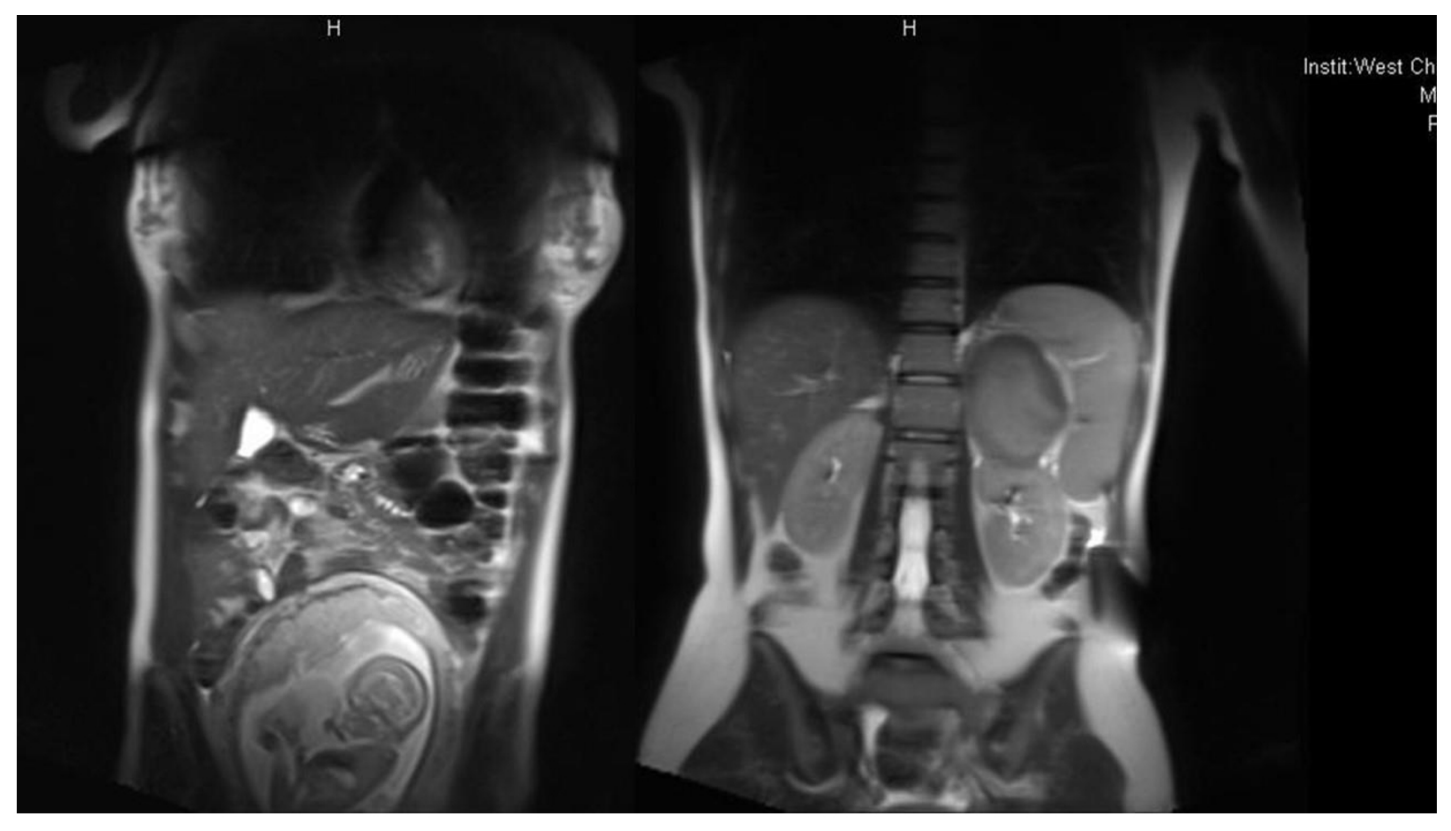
MRI of the adrenal adenoma in a woman with CS during pregnancy.
